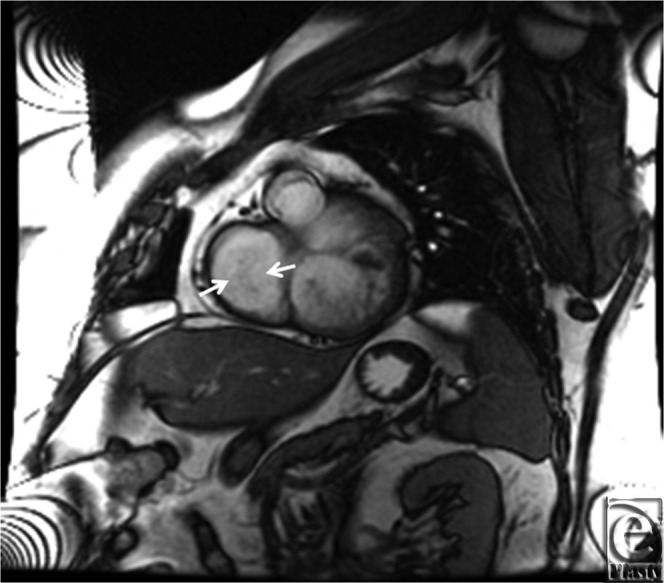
MRI cine SSFP short axis showing a bileaflet valve (white arrows) with in a morphologic left ventricle. This ventricle is noted to be anterior and supports the pulmonary circulation. MRI indicates magnetic resonance imaging.
